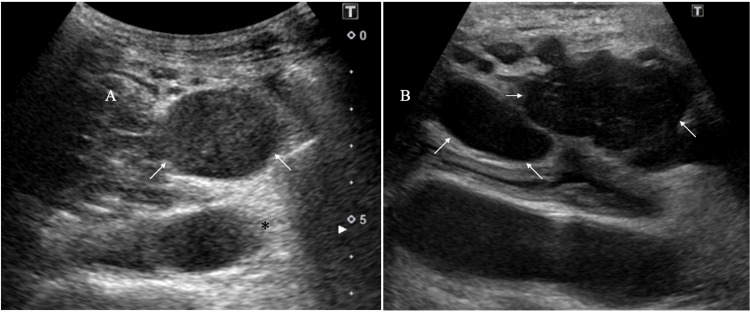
Ultrasound findings A. The target lesion (arrows) show an oval shape with clear margins, internal punctate high echoes, and enhanced posterior echoes (asterisk) B. Positron emission tomography-positive lesions (arrows) show oval or lobulated masses with very low internal echoes.
